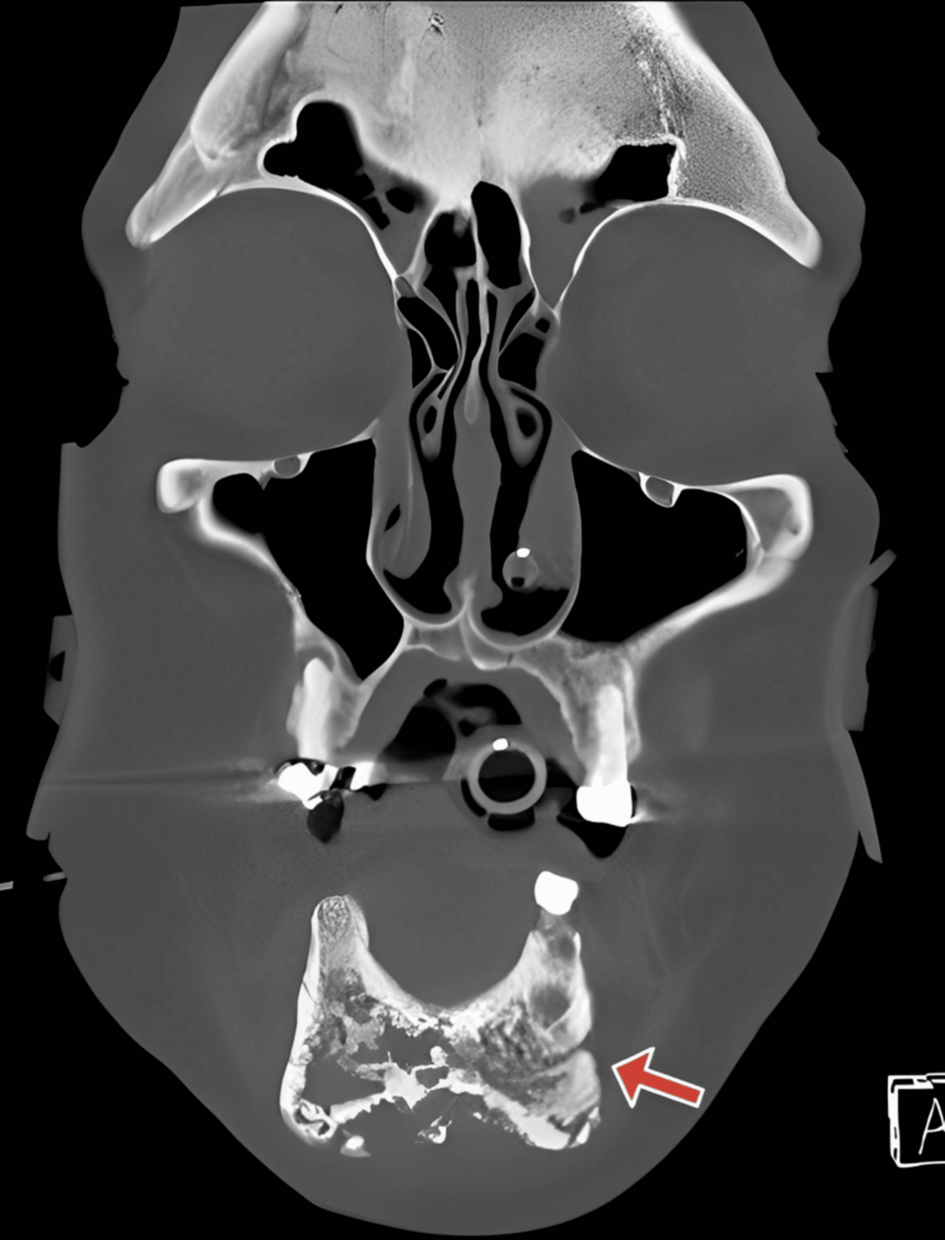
This computed tomography (CT) scan presents a coronal view of the maxillofacial region, highlighting the mandibular bone exhibiting a moth-damaged appearance (indicated by the red arrow).
